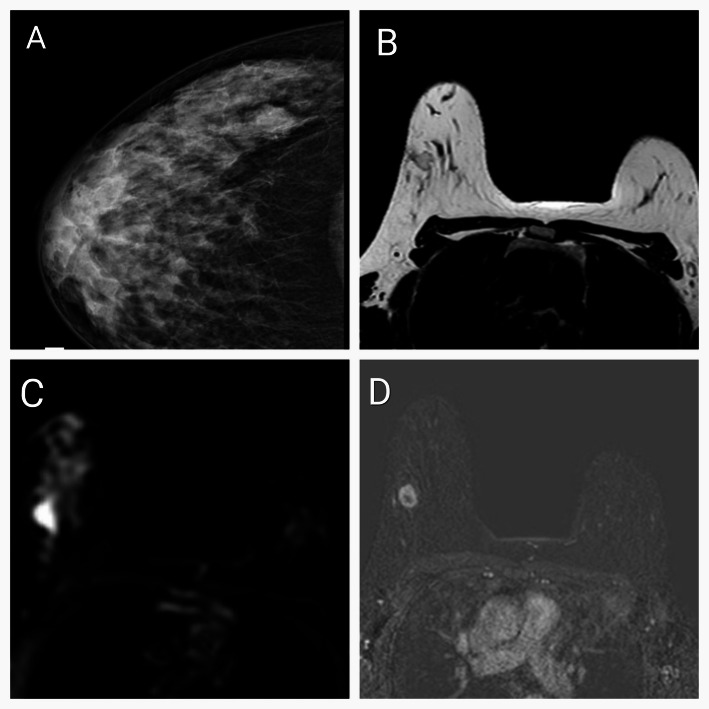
Imaging of mucinous breast carcinoma: A CC mammogram view shows an indistinct margin, high-density lesion in the right upper outer quadrant. B MRI, T2WI, the lesion shows high signal intensity (SI). C MRI, diffusion-weighted image (DWI), the lesion shows high SI on high b value. D MRI, Early subtraction image shows rapid heterogeneous enhancement of the lesion
